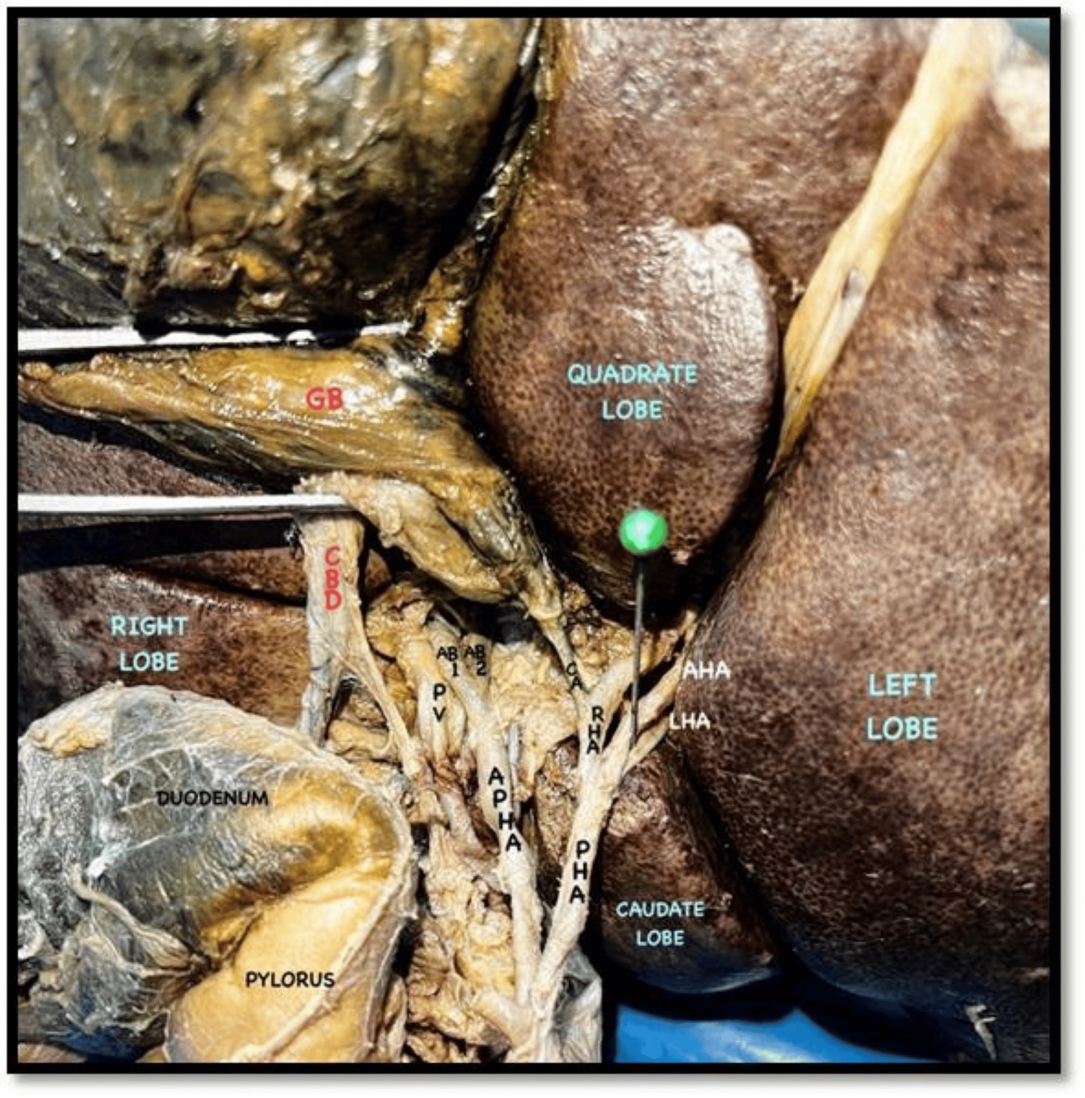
APHA and its branches (AB1 and AB2) and PHA and its branches (RHA, MHA, and LHA) (closer view) AB1, arterial branch 1; AB2, arterial branch 2; APHA, accessory proper hepatic artery; CA, cystic artery; CBD, common bile duct; CHA, common hepatic artery; CT, coeliac trunk; GB, gall bladder; GDA, gastroduodenal artery; LGA, left gastric artery; LHA, left hepatic artery; MHA, middle hepatic artery; PHA, proper hepatic artery; PV, portal vein; RGA, right gastric artery; RHA, right hepatic artery; SA, splenic artery
